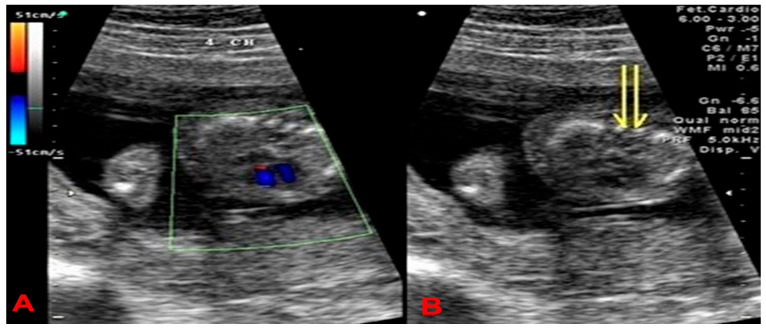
A transverse view of the fetal thorax showed a small chest, and a normal four-chamber view under color Doppler ultrasound (A) and absence of ossification of the vertebral body (two yellow arrows indicate only ossification of vertebral pedicles in the right figure, (B)).
